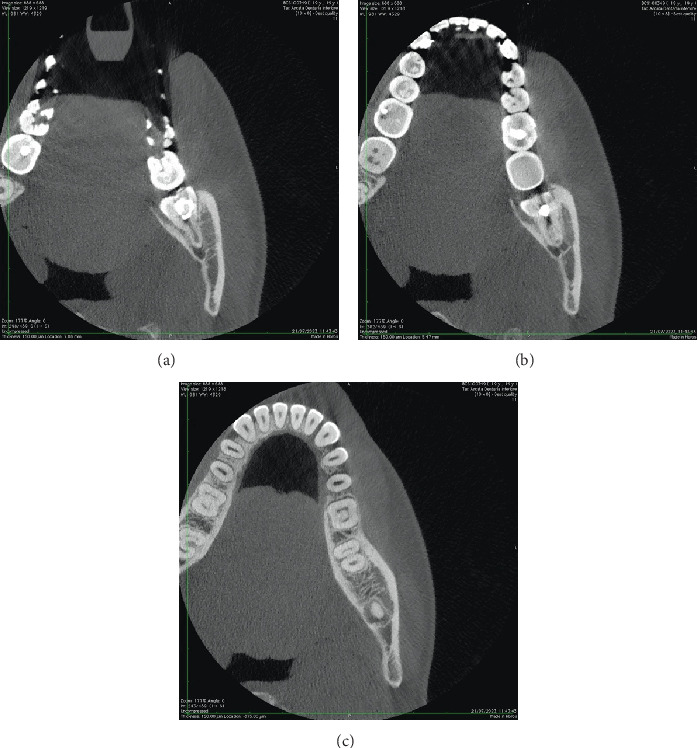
(a–c) Axial CBCT sections of the 3.8 tooth element where the continuity relationship of the root apex with the NVB can be appreciated.
